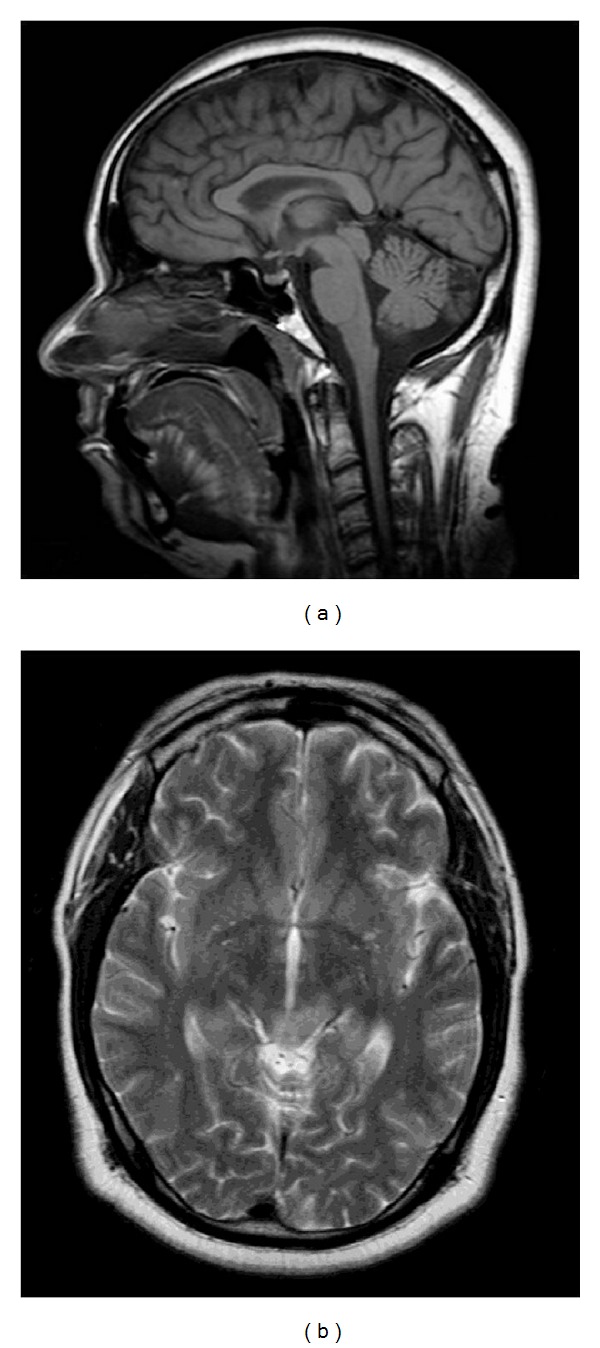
Nothnagel Syndrome. MRI images of the midbrain with enlargement of the midbrain tectum, including the quadrageminal plate, noted on sagittal T1-weighted (a) image. There is associated abnormal T2 hyperintensity seen on accompanying axial T2-weighted (b) image. These findings likely represent tectal glioma with involvement of the oculomotor nuclear complex and decussating fibers of the superior cerebellar peduncle.
